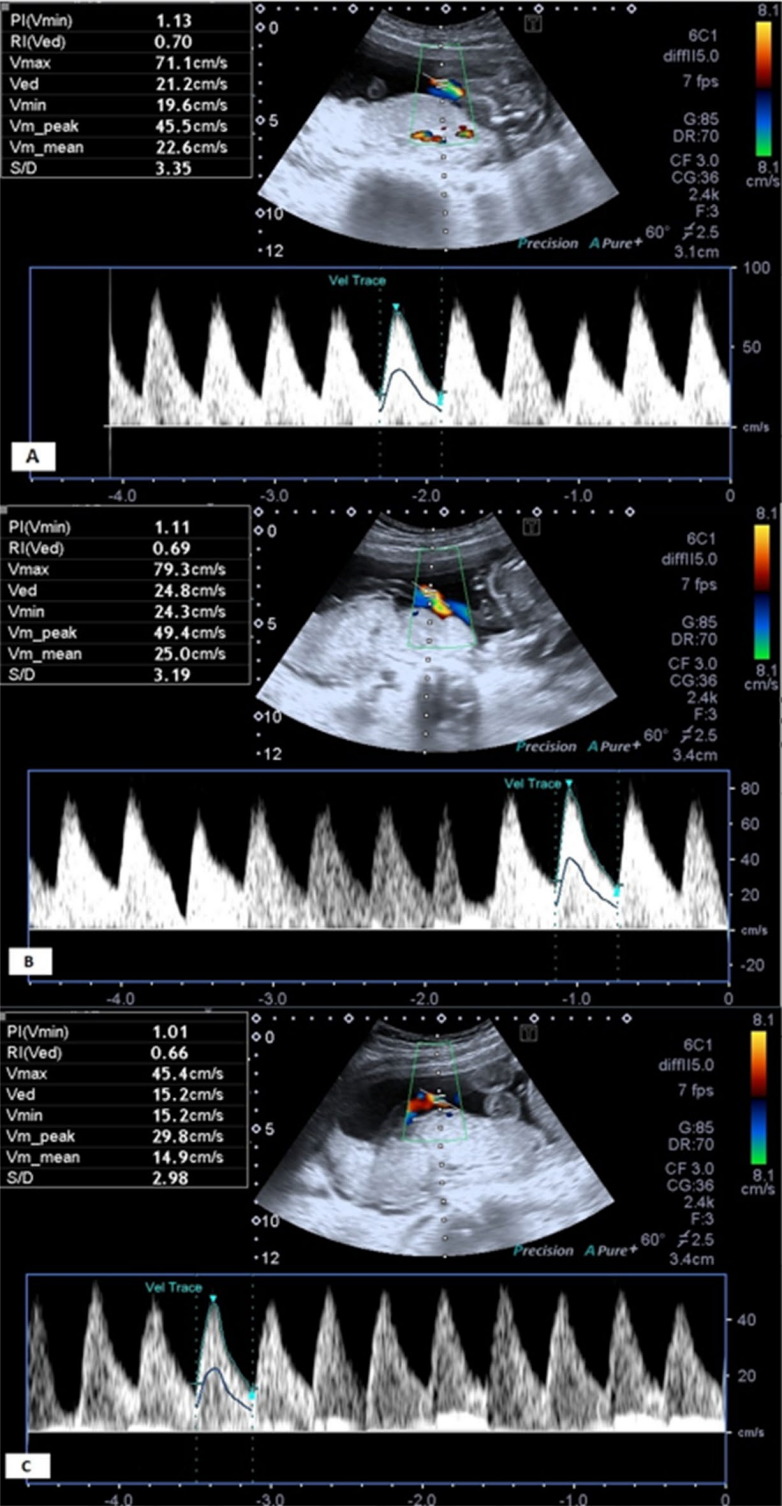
Flow patterns and Doppler ultrasound indexes of the umbilical artery from the fetal end (A), mid-portion/free loop (B), and the placental end (C).
